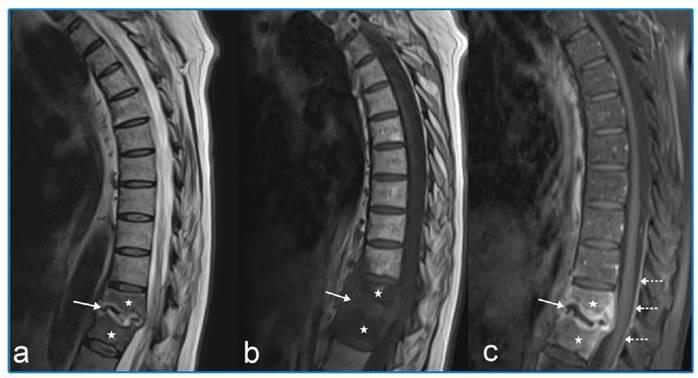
Pre-treatment MRI. Sagittal T2 WI (a): hypointense appearance are seen in the vertebra T11 and T12 (star), irregularity in the disc space, erosions in the vertebral plates, and increased intensity (arrow). T1 WI (b): marked signal losses (arrow) are seen in the vertebral bodies (star) and disc space. Contrast-enhanced T1 WI (c): contrast increases are seen in the vertebral bodies (star), disc space and vertebral plates (arrow), and posterior epidural space (dashed arrow).
